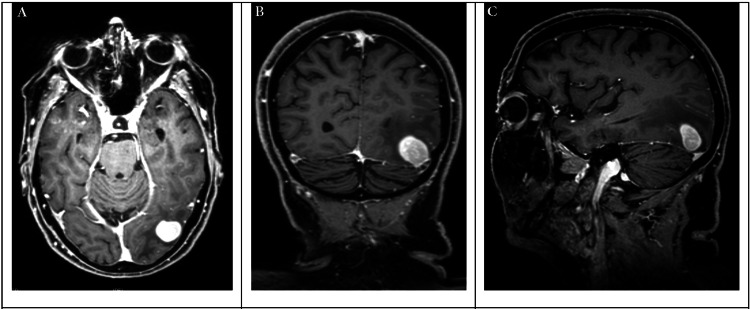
Presurgical imaging Preoperative T1-weighted head MRI images with contrast. (A) Axial, (B) coronal, and (C) sagittal view.
